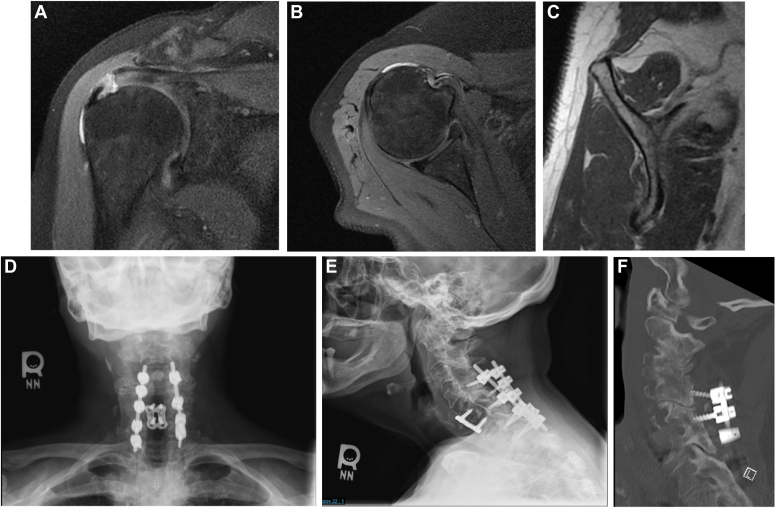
(A-C) MRI and radiographic images from clinical vignette #1. (D-F) Radiographic images from clinical vignette #1. *MRI*, magnetic resonance imaging.
